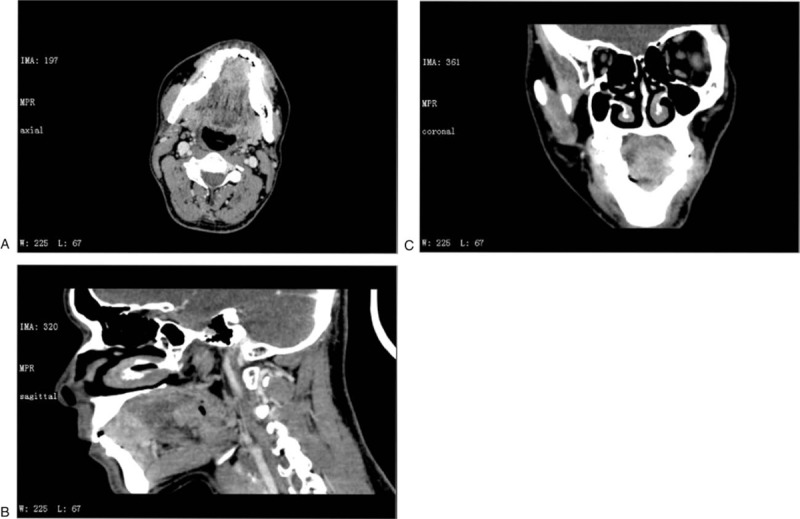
An ill-defined measuring 23 × 19 mm high density mass with altered enhanced signal entities involving the anterior 2/3rd of the tongue shown on a contrast-enhanced computed tomography. (A. axial view; B. coronal view; C. sagittal view.)
